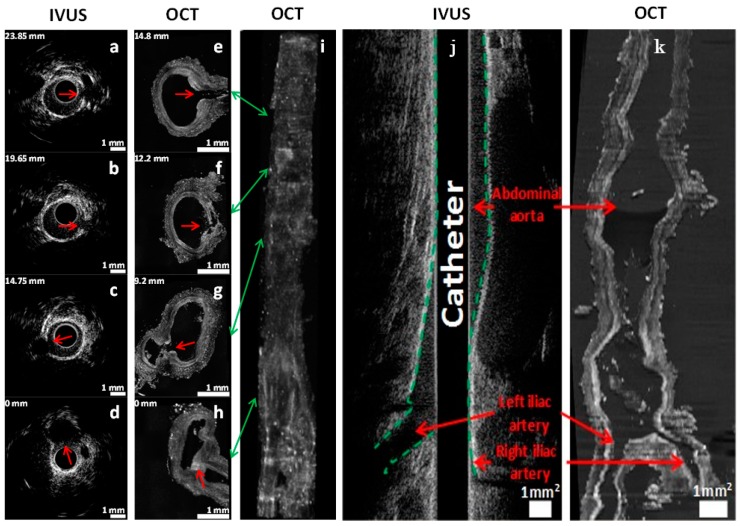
Intravascular ultrasound (IVUS) and OCT colocalization of anatomical landmarks in model 2. (a–d) In vivo IVUS cross-sectional images; (e–h) Ex vivo OCT cross-sectional images; (i) 3D reconstruction in OCT using a maximum intensity projection algorithm. Indicated numbers in mm (upper left of each image) represent the distances between the cross-section and the iliac bifurcation. The catheter was introduced in the right iliac artery, located at the bottom-right in the OCT image in (h,i). Green arrows indicate the location of the cross-section slices on 3D reconstruction. Red arrows denote side branches (anatomical landmarks) used for colocalization. Longitudinal view of the abdominal aorta and iliac arteries in IVUS and OCT imaging in model 2; (j) In vivo IVUS image of a 50 mm artery segment (green dashed lines delineates the arterial wall); (k) Ex vivo OCT image of the same segment, which shrunk to a length of 30 mm after ex vivo tissue fixation. Scale bars represent a region of 1 mm by 1 mm.
